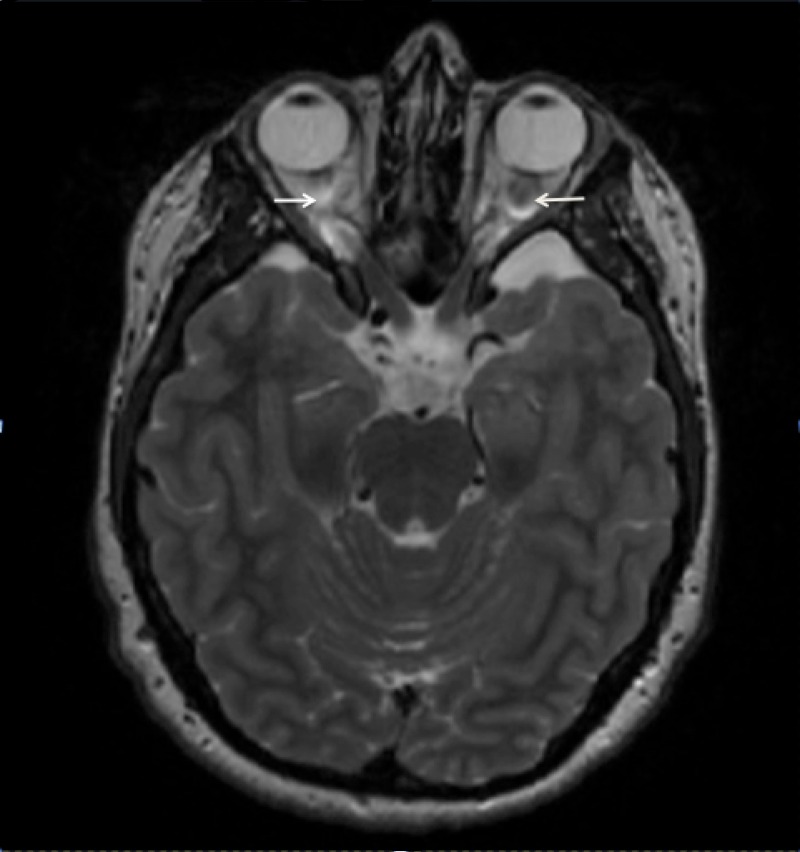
T2 weighted axial MRI through the suprasellar cistern Bilateral optic nerve enlargement consistent with optic nerve gliomas. No significant mass effect on adjacent vasculature.
